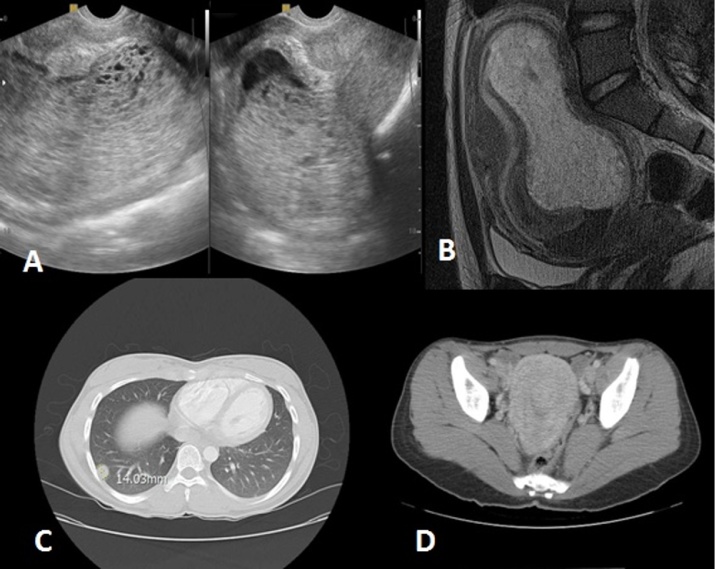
A 21-year-old woman with a molar pregnancy. (A) Sagittal and coronal transvaginal sonography images, and (B) sagittal, T2-weighted pelvic MR images showing a heterogeneous molar mass at the time of preliminary diagnosis. At follow-up, the disease progressed to choriocarcinoma. (C) Axial chest CT scan with a lung window showing metastatic nodules; (D) pelvic CT scan when choriocarcinoma was diagnosed shows a heterogeneous mass in the endometrial cavity.
